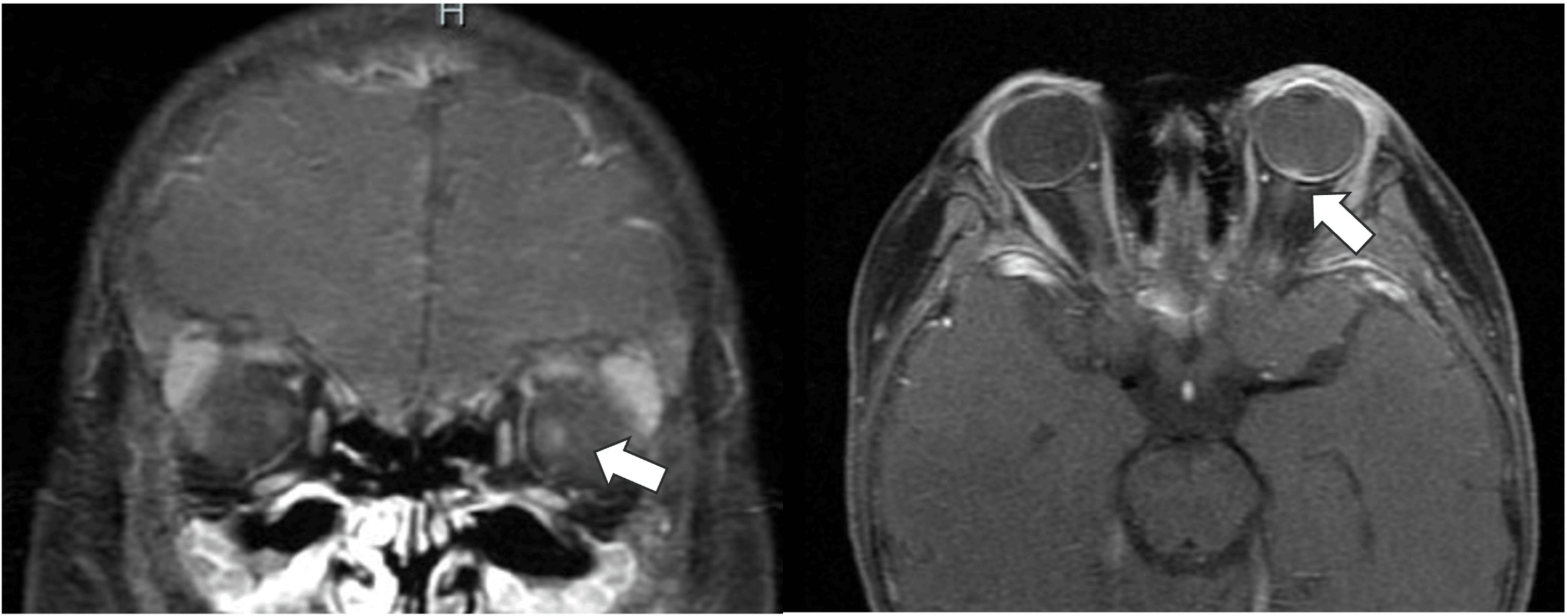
Contrast-enhanced MRI of the head Contrast-enhanced MRI after admission showed a mild contrast-enhanced effect in the left peripapillary and anterior optic nerve. There was no hyperostotic change.
